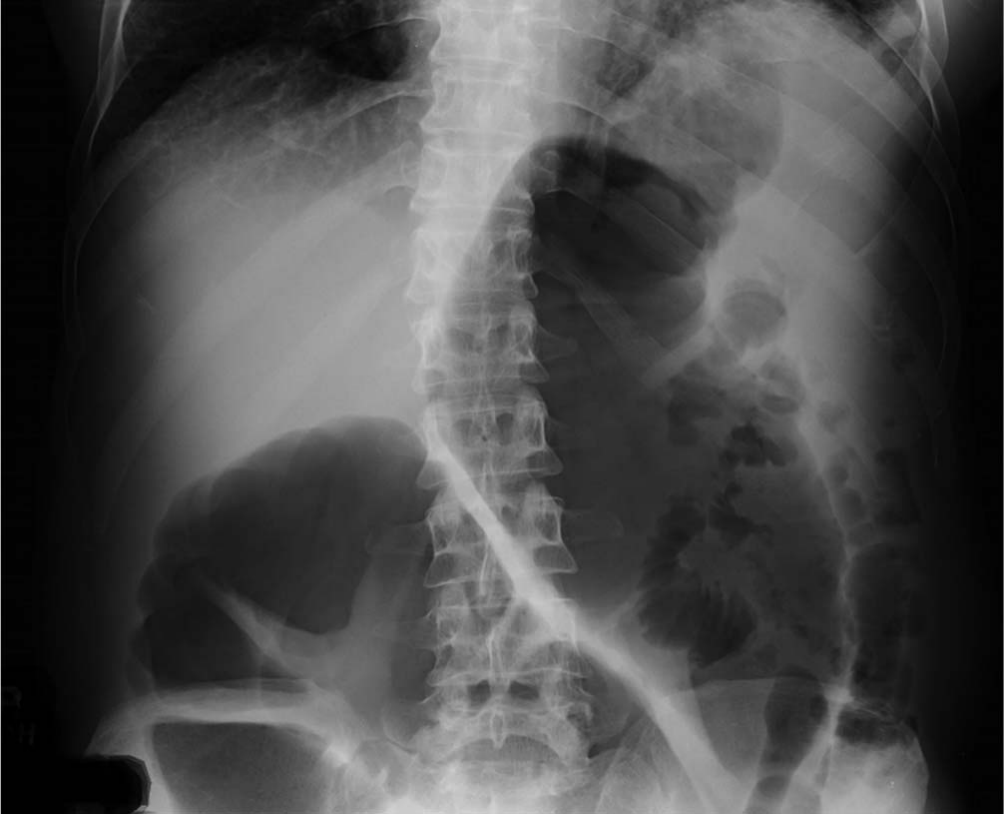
An x-ray showing diffuse marked dilatation of the ascending and transverse colon measuring up to approximately 15 cm in a patient with amyloidosis.
